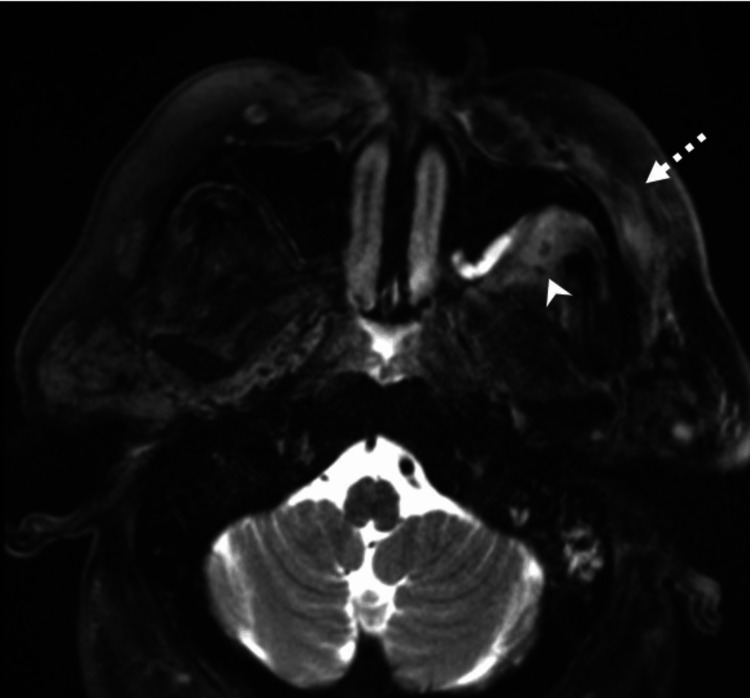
MRI of the paranasal sinuses Axial T2-weighted MRI image shows diffuse soft tissue edema involving the left buccal space (dashed white arrow), left pterygopalatine fossa, and retroantral region (white arrowhead). These findings are most consistent with cellulitis.
